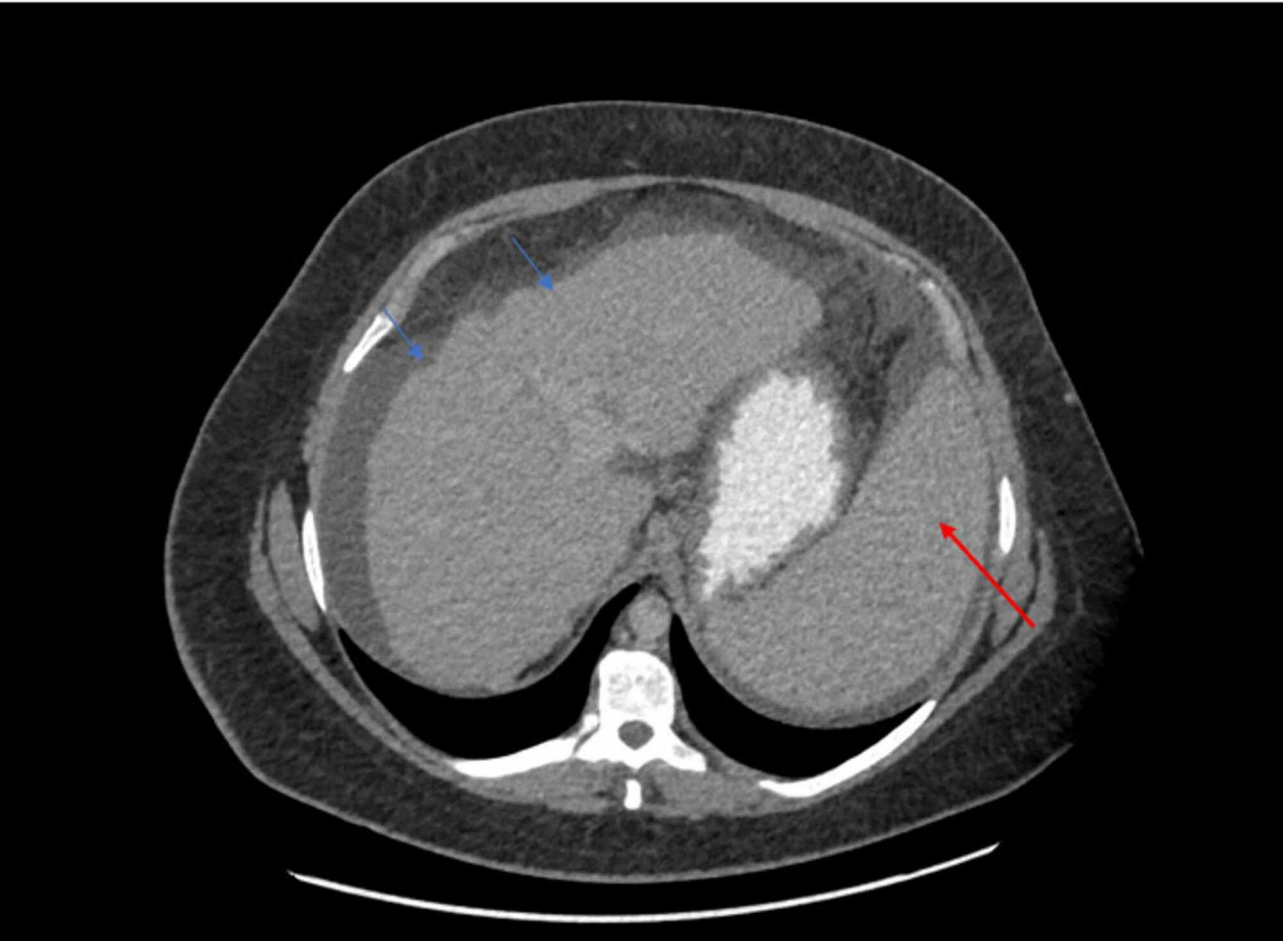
Abdominal computed tomography (CT) showing a nodular contour of the liver concerning for liver cirrhosis (blue arrowhead) and splenomegaly (red arrowhead)
